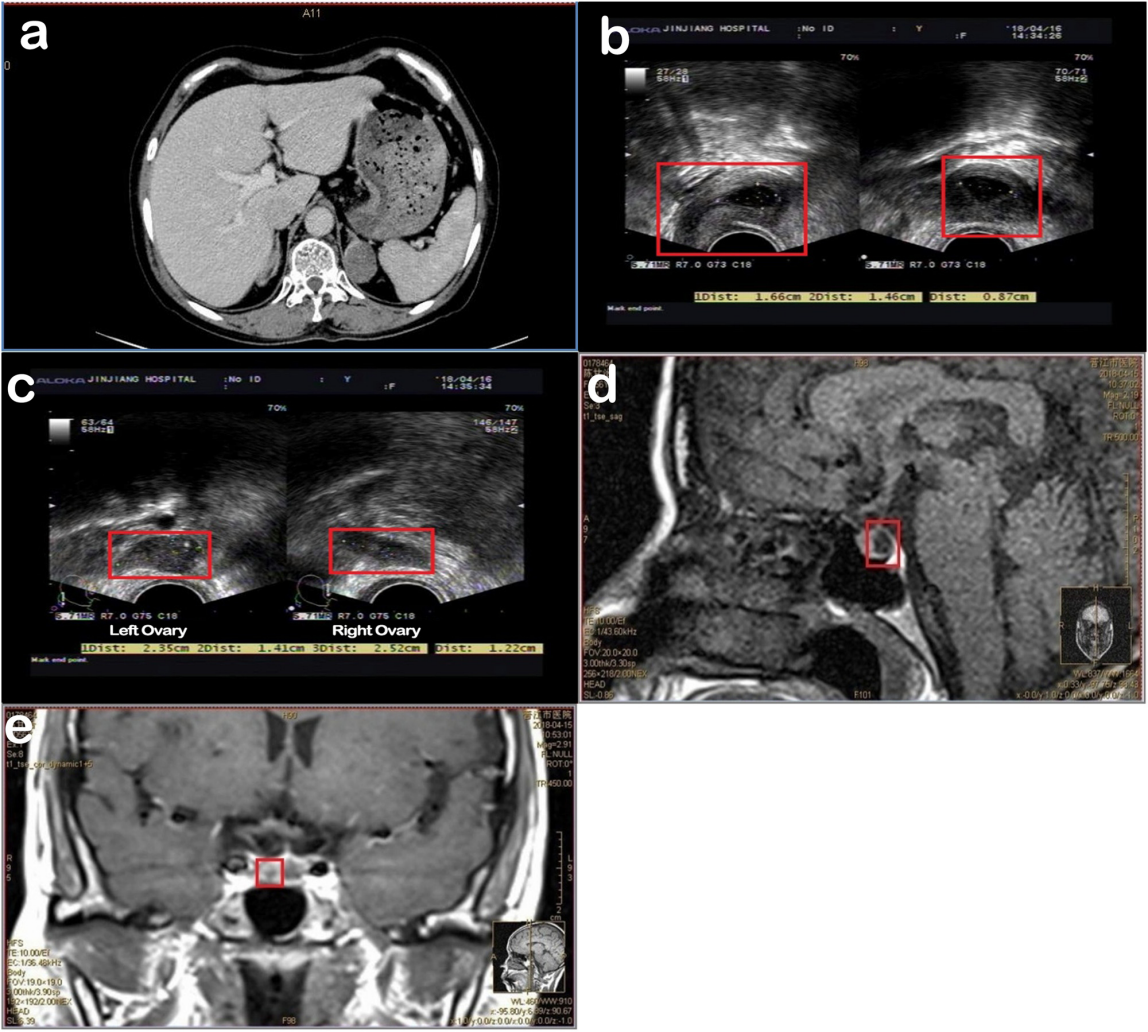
(a) Non-enhanced and enhanced CT scanning of adrenal gland; (b) transvaginal ultrasound of the female reproductive system 1, the female reproductive system was examined using transvaginal ultrasonography, and the results showed that the uterus was small (2.6 cm × 2.6 cm × 2.1 cm) and that the shape was regular; (c) transvaginal ultrasound of the female reproductive system 2, both the ovaries were small, the size of the left ovary was c. 2.5 cm × 1.2 cm, and the size of the right ovary was c. 2.4 cm × 1.4 cm. This needs to be differentiated from the ovaries of postmenopausal women because the vulva of this patient also shows undeveloped performance, which is different from the vulva of postmenopausal women, the reason for the small ovaries of this patient might be caused by pathological factors. (d) MRI1 of pituitary gland; (e) MRI 2 of pituitary gland.
